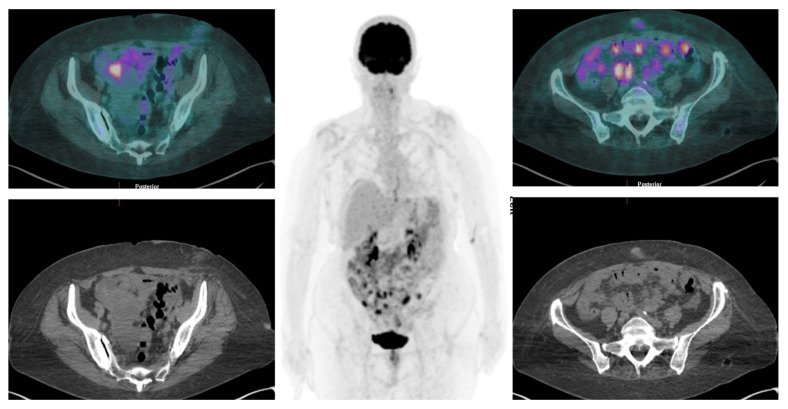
[^18^F]FDG PET/CT detects multiple nodular hypermetabolic lesions in the abdominopelvic region, suggestive of peritoneal carcinomatosis.
